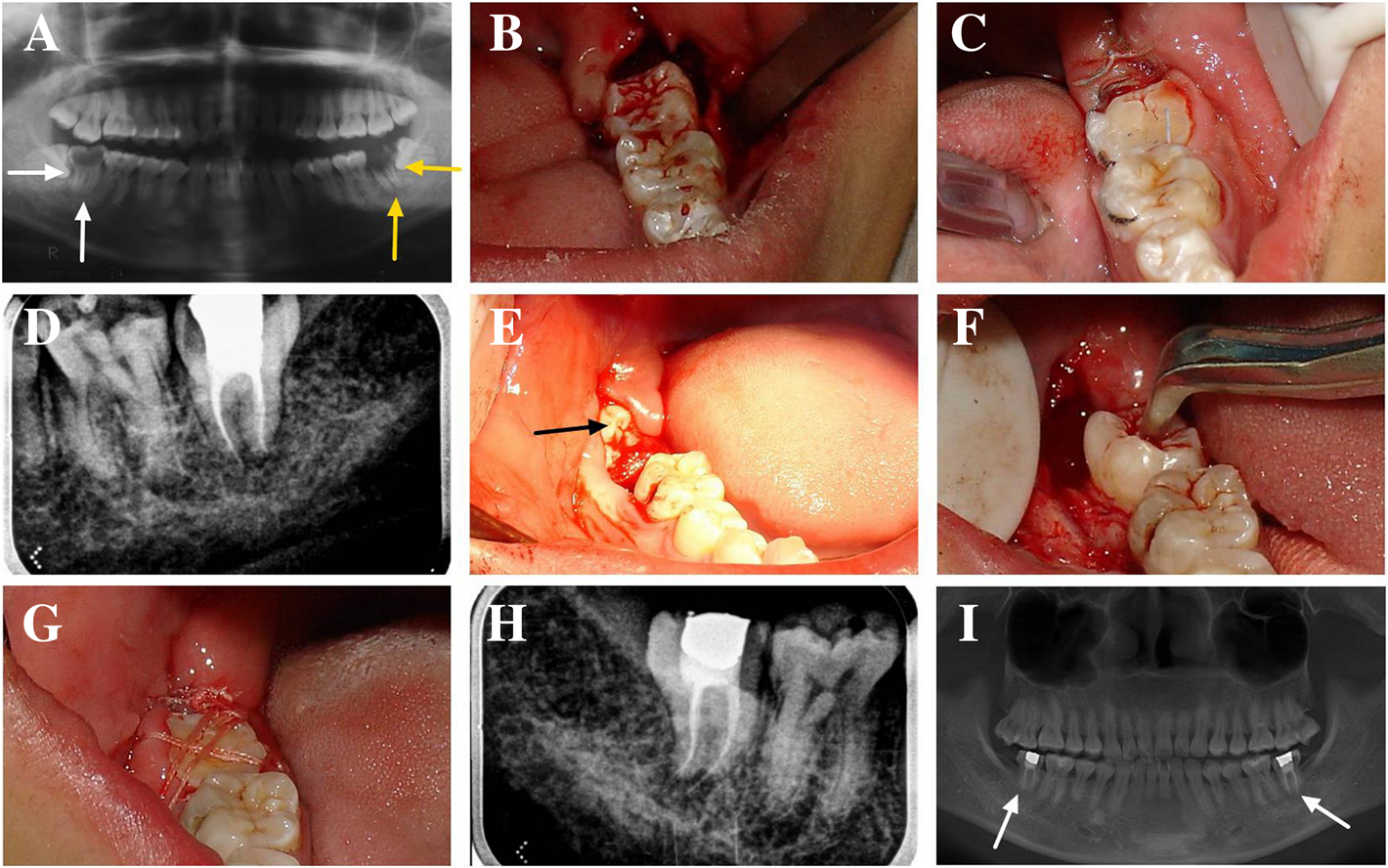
Course of treatment and long-term follow-up in a 24-year-old patient who had 2 third molars transplanted. The #38 tooth replaced the #37 tooth, and the #48 tooth replaced the #47 tooth. a A panoramic radiograph revealed severe caries of the left and right mandibular second molars (#37, #47). Radiolucent areas were visible at the apices of the #37 and the #47 teeth. Neither could be restored, and both were extracted. b The #38 tooth was transplanted into the #37 position after being rotated 180°. c The #38 tooth was fixed with wire after occlusal adjustment. d An intraoral radiograph of the #38 tooth immediately after endodontic treatment 4 weeks after transplantation. e An intraoral photograph of the #48 tooth before transplantation. f The #48 tooth was transplanted into the #47 position after being rotated 180°. g The #48 tooth was fixed via suturing because the #48 tooth extended too far. After occlusal adjustment, the edge of the #48 tooth was at the level of the gingiva, and it was difficult to fix with wire. h An intraoral radiograph of the #48 tooth immediately after endodontic treatment 4 weeks after transplantation. i Panoramic radiograph after 7 years of follow-up. The radiolucent areas at the apices of the #38 and the #48 teeth have disappeared
